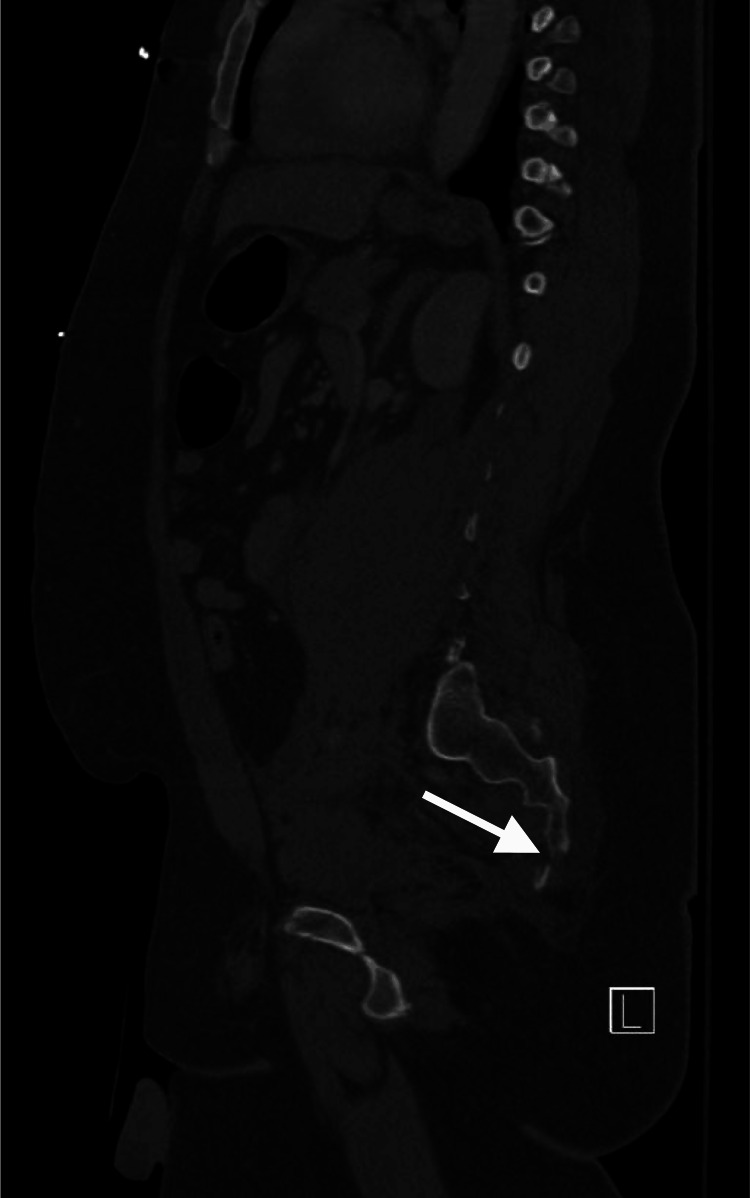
CT scan of the pelvis shows a distal sacral fracture.
